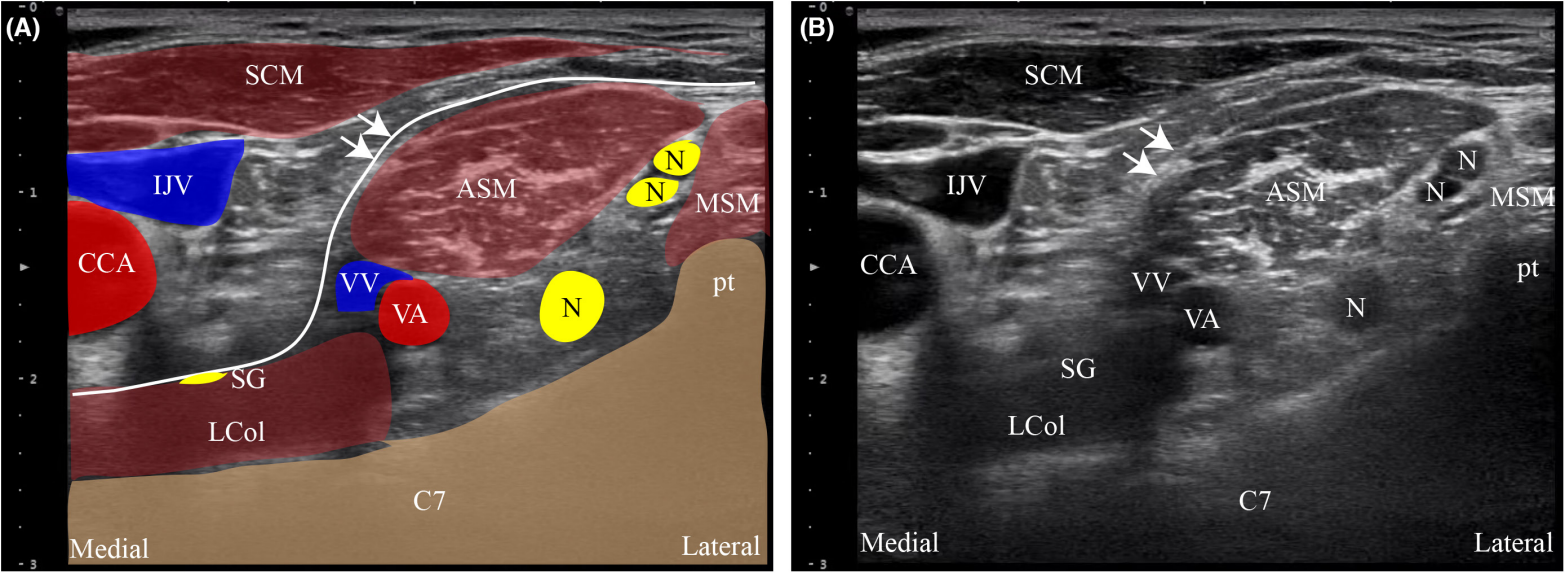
Short axis sonogram at C7. (A, B) The transducer is moved slowly laterally and cephalad until the characteristic posterior tubercle of C7 appears in the image. The solid arrow shows the anterior vertebral fascia. The stellate ganglion is located on the deep surface of the anterior fascia and the surface of the longus colli muscle. ASM, anterior scalene muscle; CCA, common carotid artery; IJV, internal jugular vein; Lcol, longus colli muscle; MSM, middle scalene muscle; N, nerve; pt, posterior tubercle; SCM, sternocleidomastoid muscle; SG, stellate ganglion; VA, vertebral artery; VV, vertebral vein.
